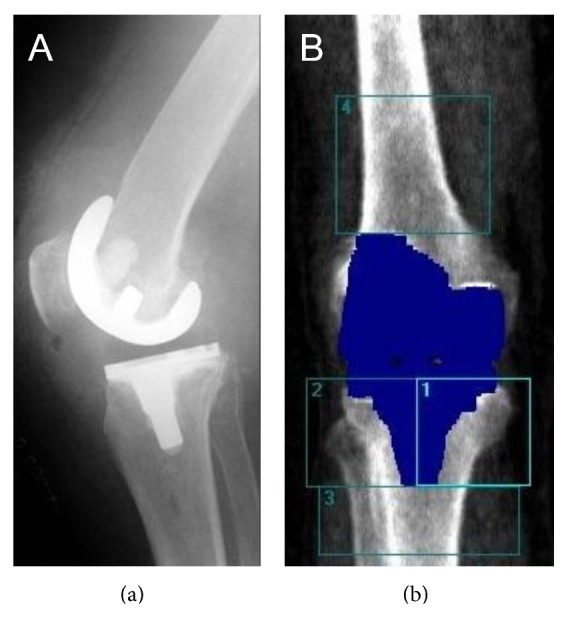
(a) Lateral X-ray scan of the implant and (b) anterior-posterior dual-energy X-ray absorptiometry scan with the periprosthetic regions of interest (ROI) for analysing bone mineral density of the tibia (1 = ROI-T1; 2 = ROI-T2; 3 = ROI-T3) and femur (4 = ROI-F4).
